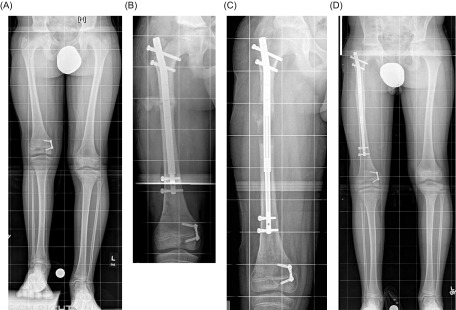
Clinical case shows normal healing. (A) Preoperative anteroposterior (AP) view full length standing radiograph of a patient with a limb length discrepancy. (B) AP view radiograph of the right femur after insertion of PRECICE. (C) AP view radiograph of the right femur after distraction. (D) Final AP view full length standing radiograph shows that the right femur healed after the lengthening goal was achieved. Figure used with permission (© 2016, Rubin Institute for Advanced Orthopedics, Sinai Hospital of Baltimore).
